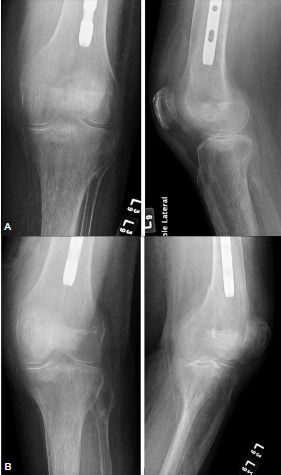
(A) Orthogonal radiographs (two-view) of one “critical miss” that was initially unidentified on orthogonal radiographs and then later identified as requiring operative management with the supplement of oblique radiographs. Seen to have a distal femoral metaphysis fracture. (B) Oblique radiographs (four-view component) of one “critical miss” that was not identified on orthogonal radiographs and then later identified as requiring operative management with the supplement of oblique radiographs. Seen to have a distal femoral metaphysis fracture.
